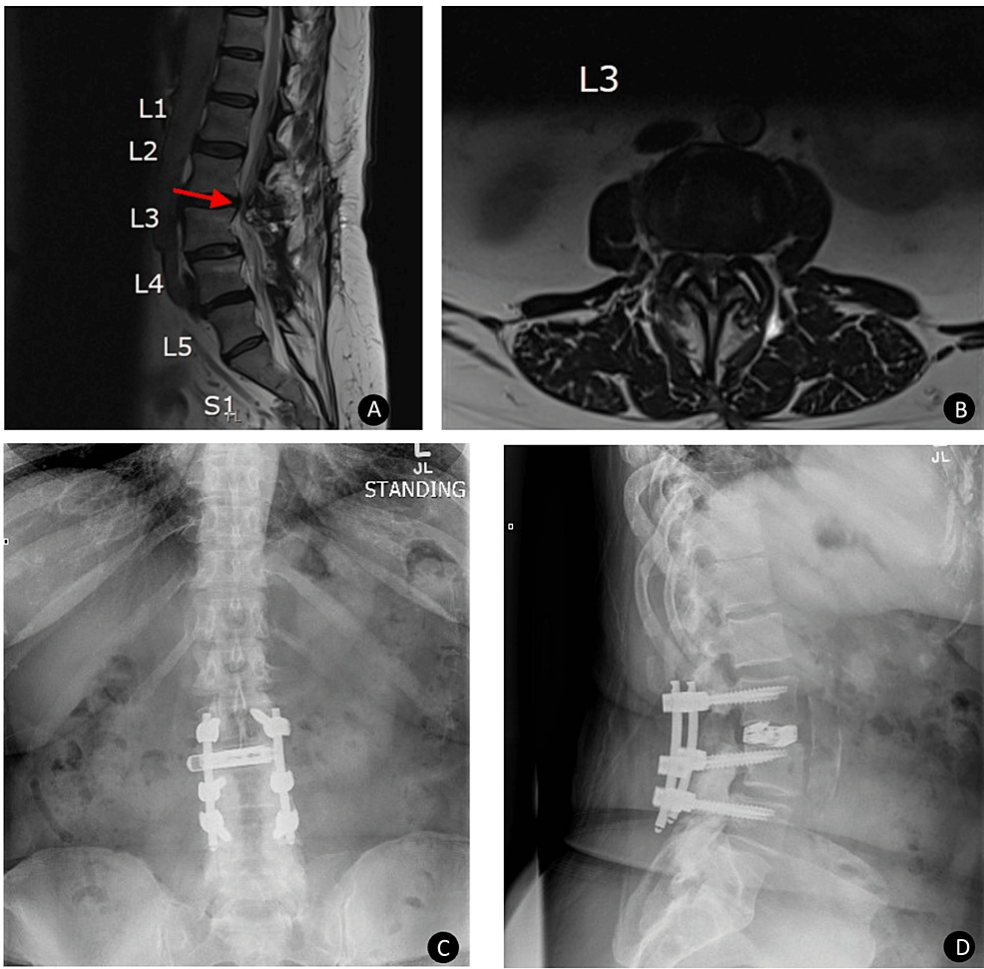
Case 2 postoperative radiographic evaluation of dual expandable spacer and screw placement A 70-year-old female presented with lumbar radiculopathy and low back pain. Preoperative sagittal (A) and axial MRI (B) showing postsurgical changes at L3-L4 from prior surgery with severe central canal stenosis at L2-L3 related to degenerative disc disease and facet arthropathy (indicated by the red arrow). Postoperative AP (C) and lateral (D) x-ray images show stable and intact posterior lumbar decompression and fixation from L2-L4 with intact interbody spacer and maintained disc space height.
